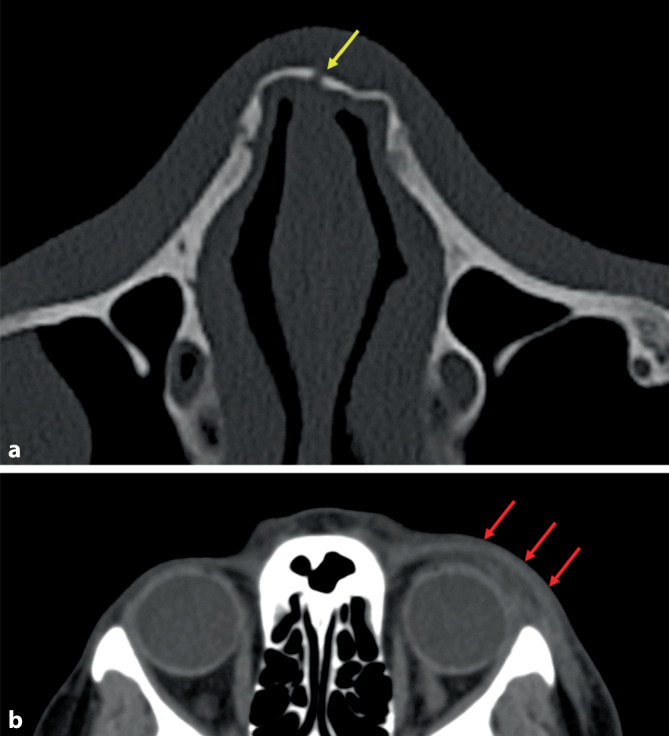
Klinische Computertomograhphie (CT), Nasenbeinfraktur (a, *gelber Pfeil*) und Monokelhämatom links (b, *rote Pfeile*) nach Faustschlägen gegen das Gesicht (unterschiedliche Patienten)
